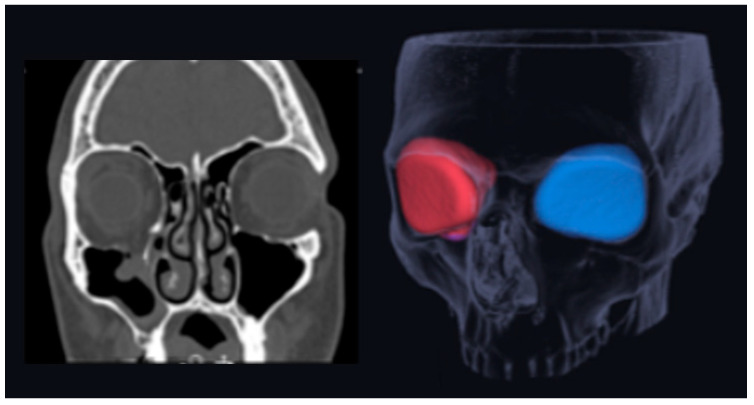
Orbital volume is measured from CT scans with an automated segmentation tool in which a virtual triangle mesh expands from a starting point in the bony orbit to another on the other side. The volume of the fractured orbit (red) is compared to the intact (blue) one to obtain the relative orbital volume change.
